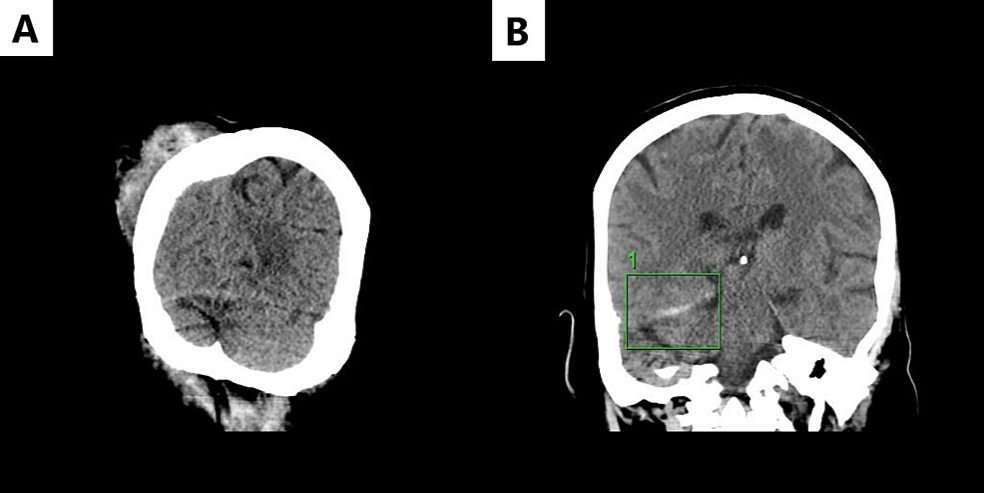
(A) Initial CT after fall showing large posterior paramedian subgaleal hematoma; (B) initial coronal CT showing small 5 mm subdural hematoma along the right tentorium CT: computer tomography
